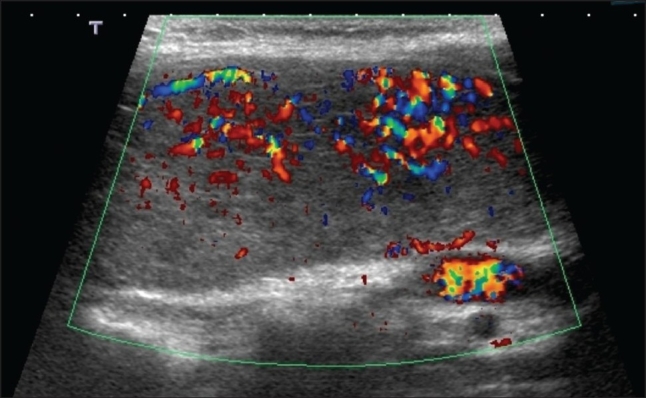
Ultrasound of the thyroid showing an intense vascularity on color Doppler that is consistent with an “inferno”.
